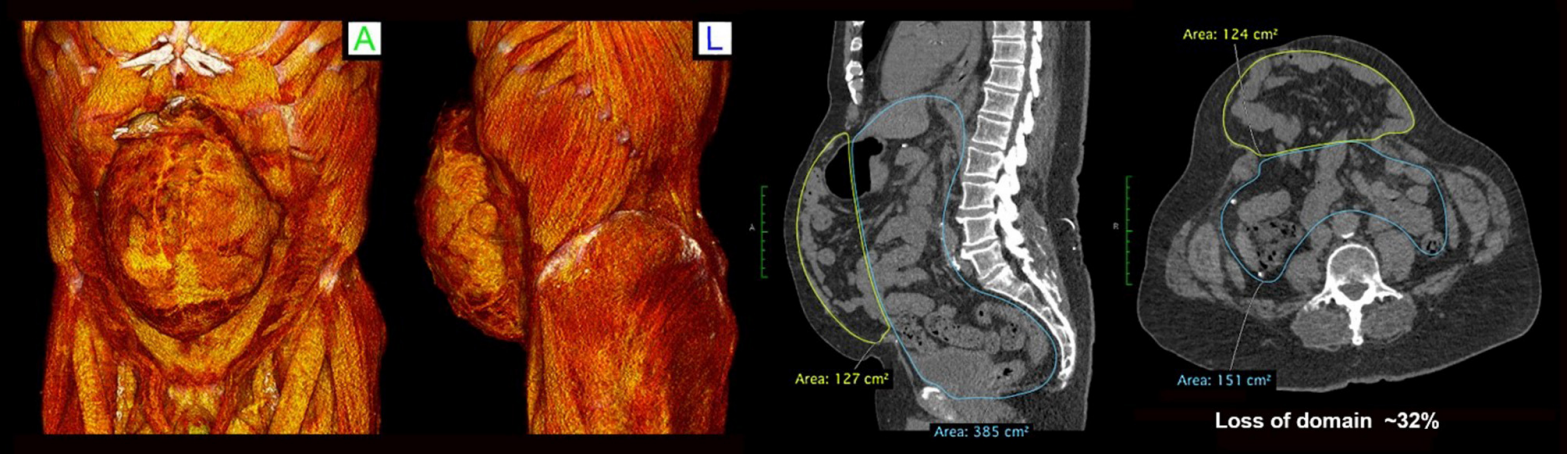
Baseline CT images demonstrating large incisional hernia post 2 open AAA repairs and two subsequent open incisional hernia repairs. Loss of domain is calculated at 32%.
